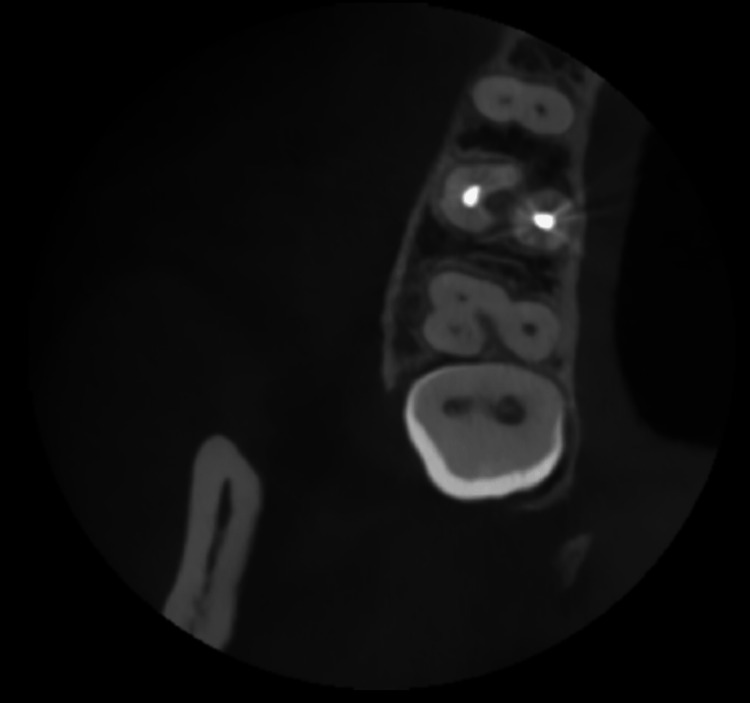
Postoperative CBCT imaging. CBCT, cone-beam computed tomography
